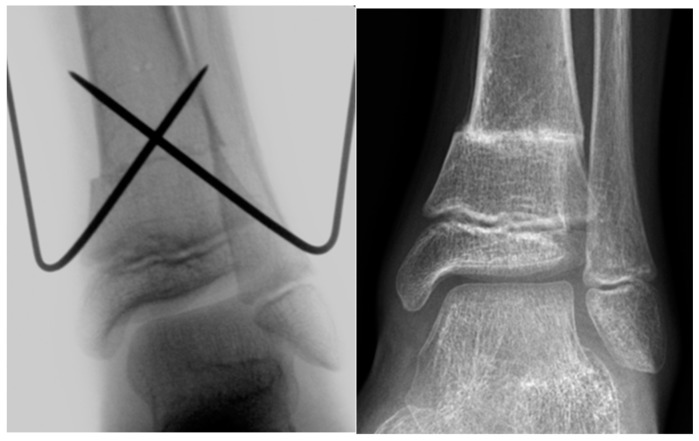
Intraoperative X-ray image and X-ray image obtained at 2 months after SMO fixed with K‑wires in an 11-year-old male patient with increased external torsion of the tibia.
